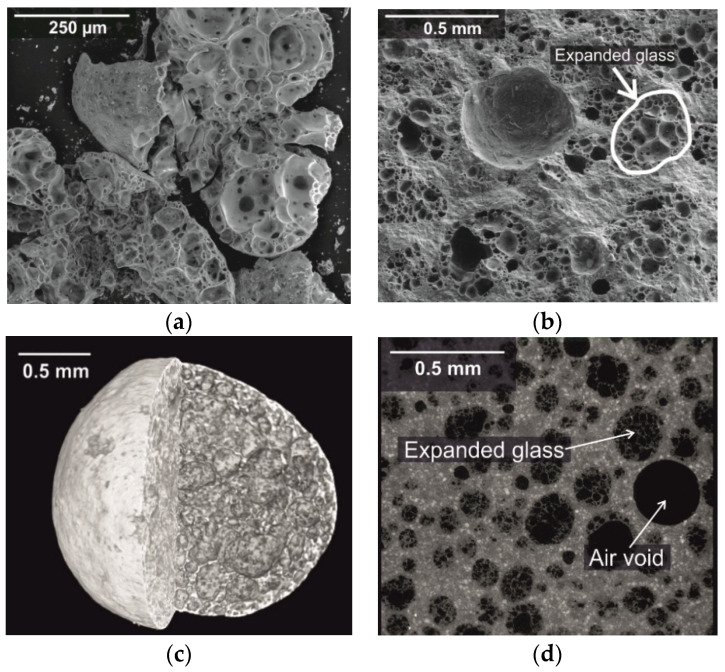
SEM images (SE mode; low vacuum, 50 Pa, 15 kV): (a) fractured expanded glass grain and (b) expanded glass embedded in UHPC on a fractured surface. CT images: (c) surface and internal structure of expanded glass, spatial resolution 1 µm, and (d) sectional view of the material structure, spatial resolution 1.5 µm.
